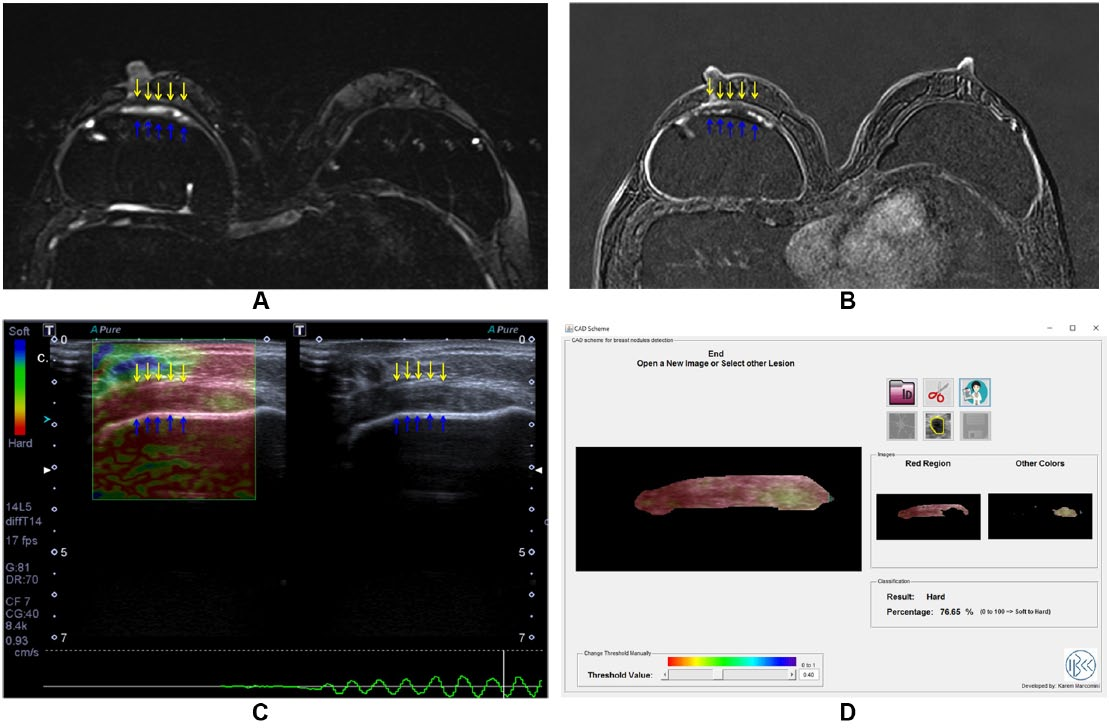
A 58-year-old woman with breast augmentation surgery 10 years ago. (A) Axial T2-weighted sequence with silicone suppression presents collection between the fibrous capsule (yellow arrows) and the elastomer of the implant (blue arrows). (B) In the dynamic sequence, there is enhancement of this collection corresponding to solid content. (C) By elastography, it is observed to correspond to hard lesion. (D) Elastography report.
